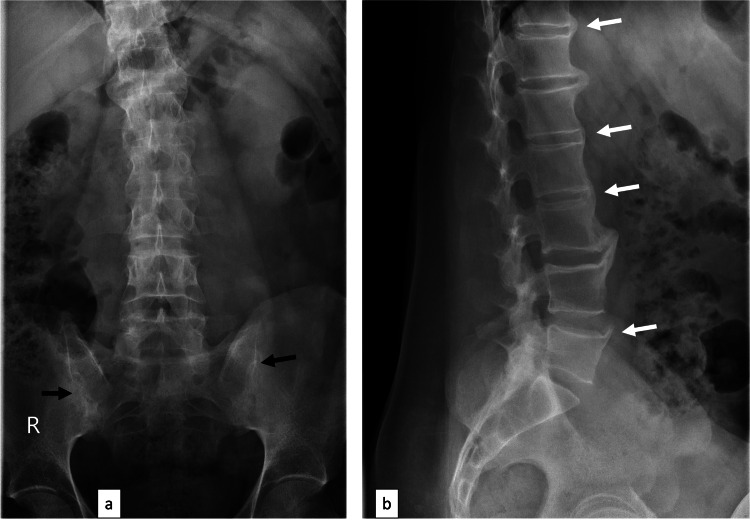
X-rays of the lumbosacral spine (a) anteroposterior and (b) lateral view showing sacroiliitis grade 3 bilaterally (black arrows) and lumbar syndesmophytes (white arrows) confirming the diagnosis of ankylosing spondylitis.
